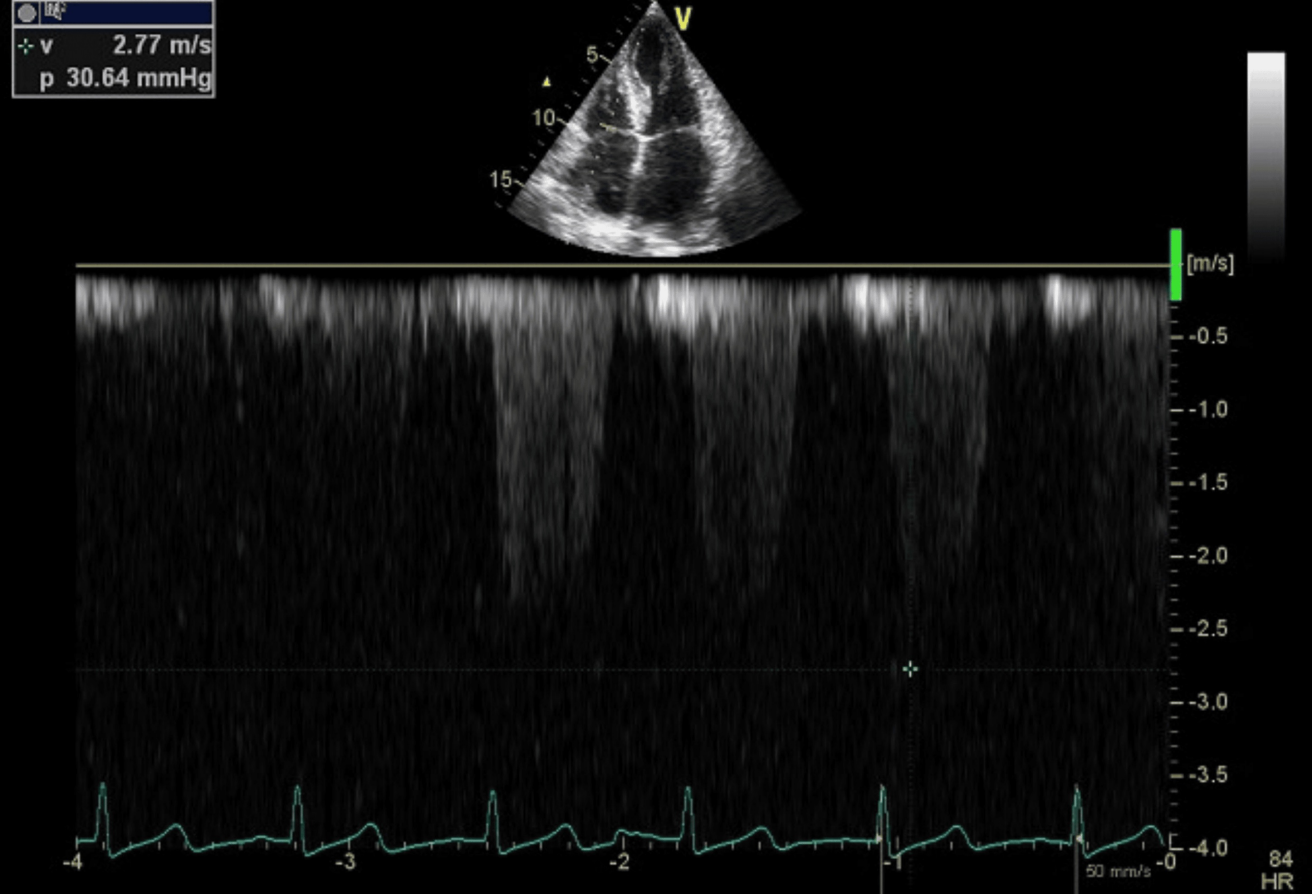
Continuous wave TTE at the LVH and mitral valve showing gradient of 30 mmHg after AVF closure TTE: transthoracic echocardiography, AVF: arteriovenous fistula, LVH: left ventricular hypertrophy
